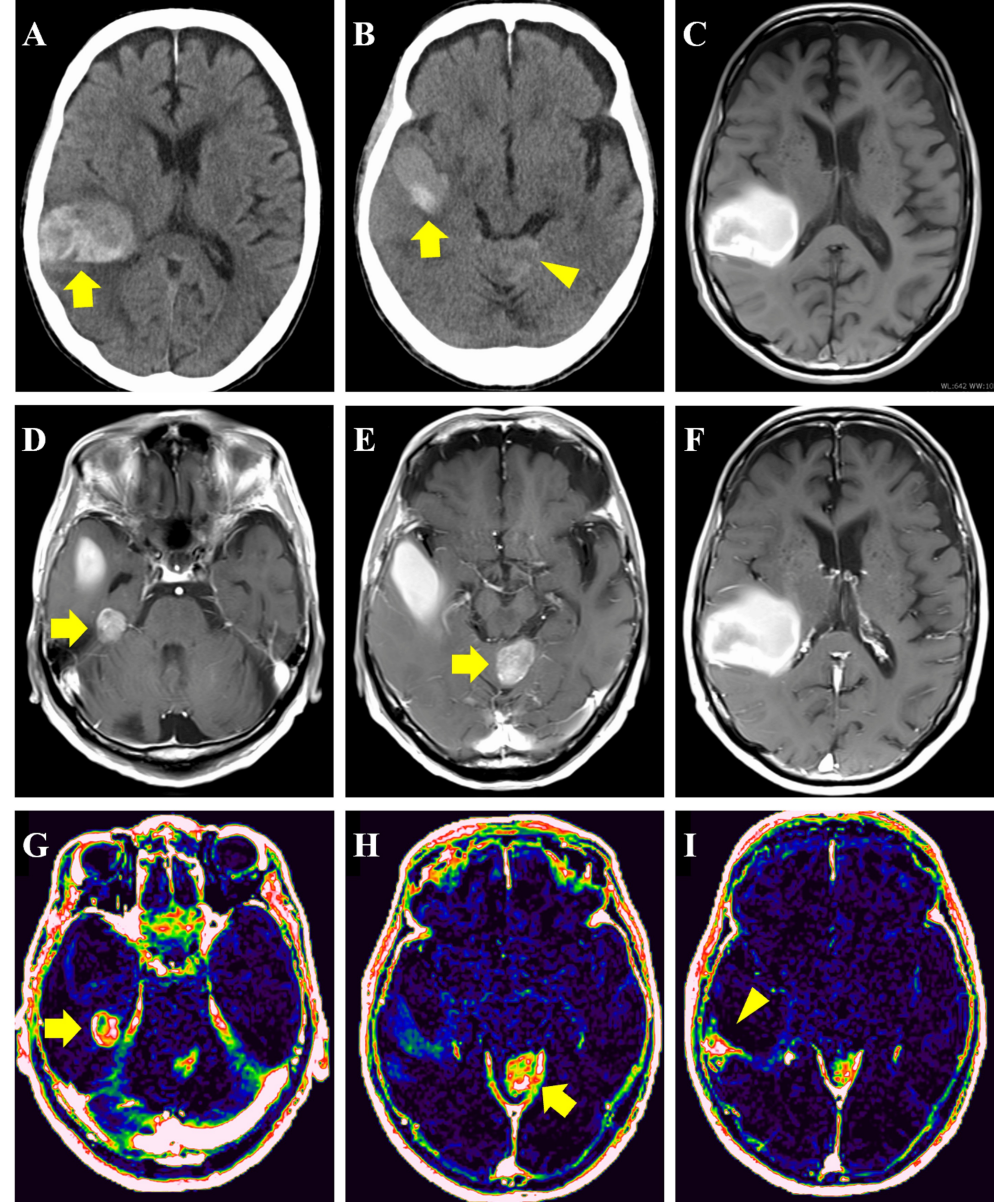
Radiological findings at disease onset A, B: Head CT showing subcortical hemorrhage (arrows) and a faint high-density area suspicious for tumor (arrowhead). C: T1-weighted MRI; D-F: Gadolinium-enhanced T1WI. D, E: Well-defined enhancing lesions (arrows). F: No enhancement. G-I: DCE-MRI–derived Ve maps. G, H: Elevated perfusion in enhancing lesions (arrows). I: Additional perfusion elevation (arrowhead) without enhancement, suggestive of another neoplastic lesion. CT, computed tomography; T1WI, T1-weighted image; DCE-MRI, dynamic contrast-enhanced magnetic resonance imaging; Ve, extravascular extracellular space volume per unit tissue volume.
